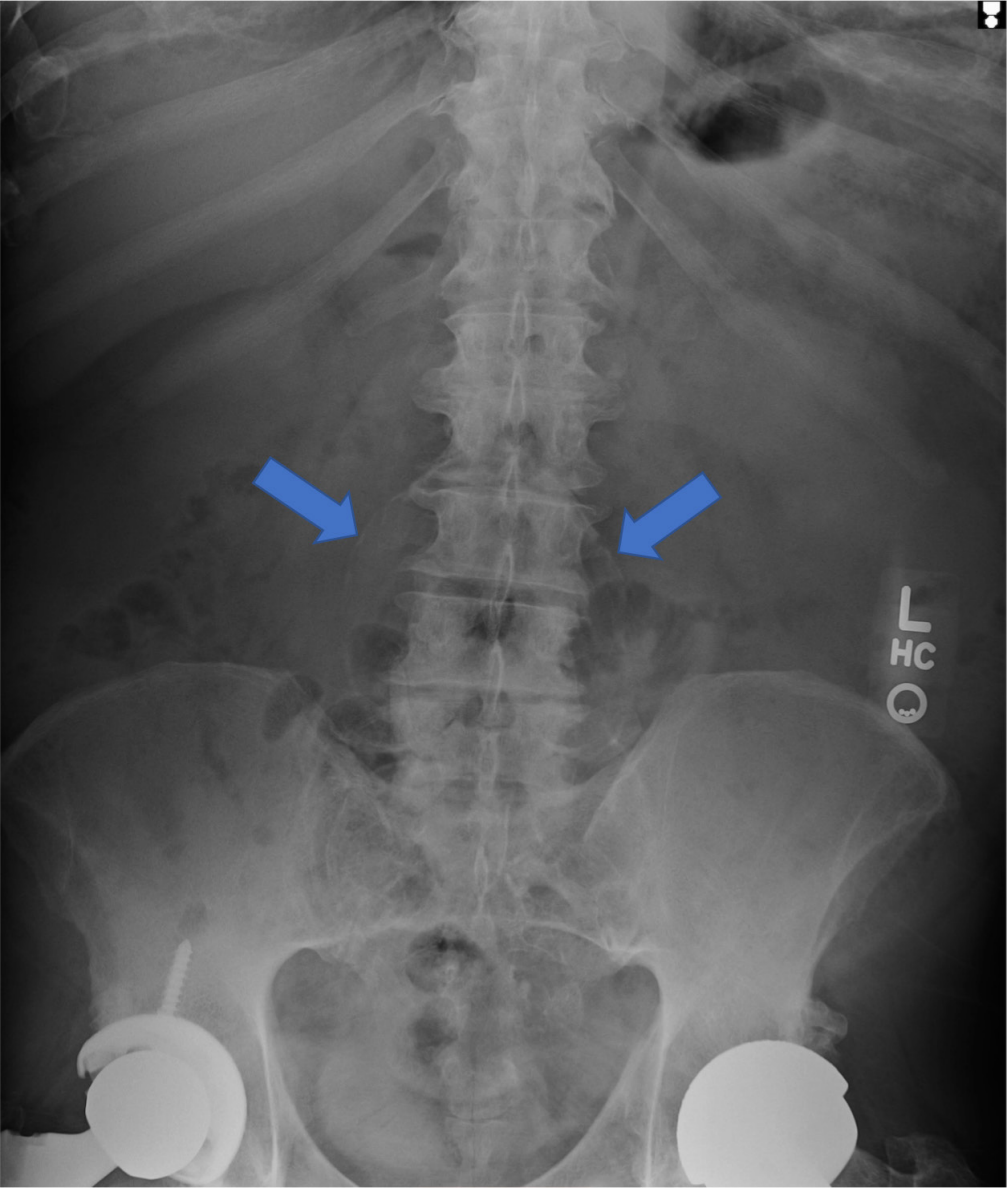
Anterior–posterior radiograph of lumbar spine demonstrating calcified outline of abdominal aortic aneurysms (blue arrows).
